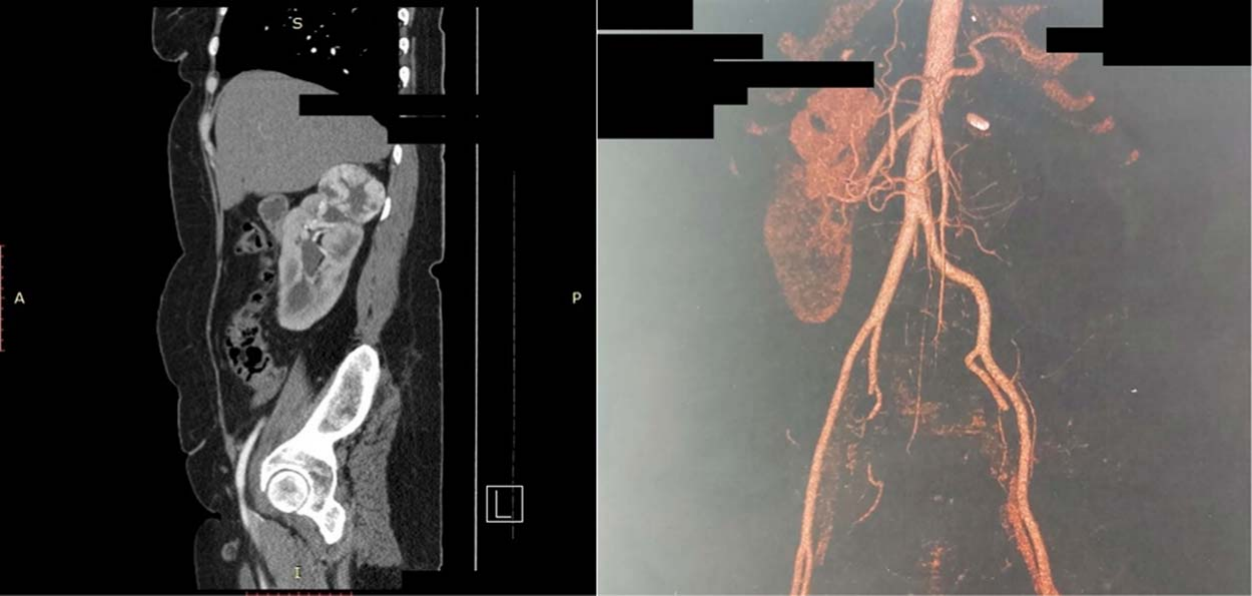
Preoperative contrast Multislice computed tomography.
